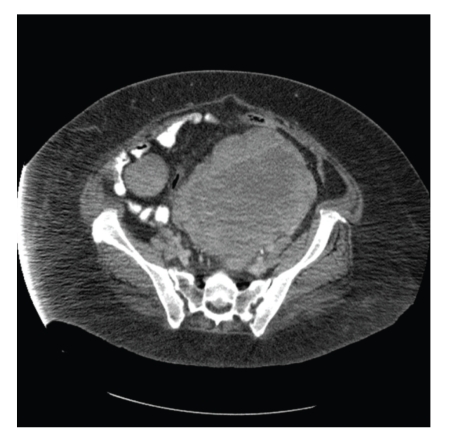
CT of the pelvis with large pelvic mass.
